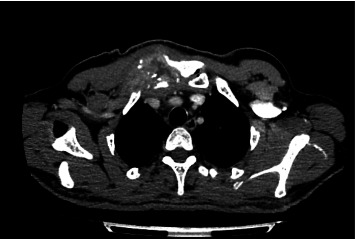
Contrast-enhanced thoracic CT scan demonstrating an expansile lytic lesion with periosteal reaction, associated with a soft tissue lesion with central necrosis and extension to the skin. The lesion involves the manubrium sterni, sternoclavicular joint, and right clavicle. Magnification: Not applicable for CT scan (displayed at standard radiological window).
